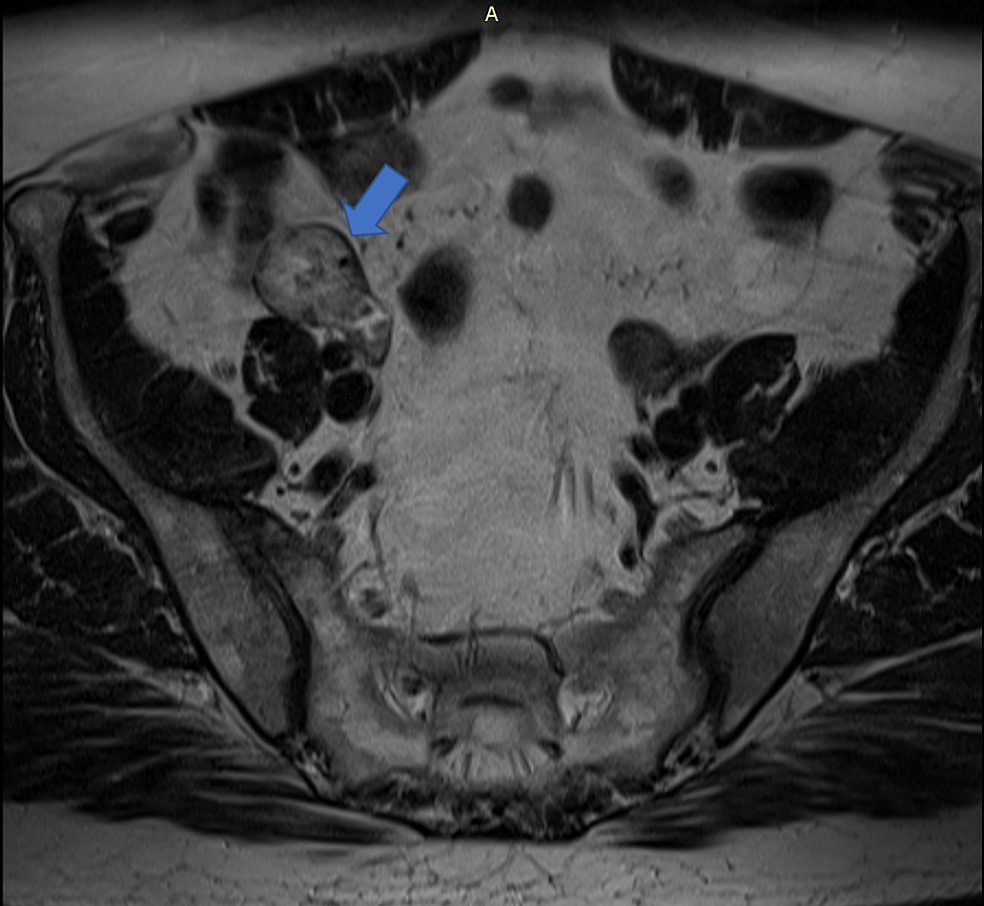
Pelvic magnetic resonance imaging A solid mass measuring 37 × 2 mm was relatively well defined in the right ovary (T2) (blue arrow).
